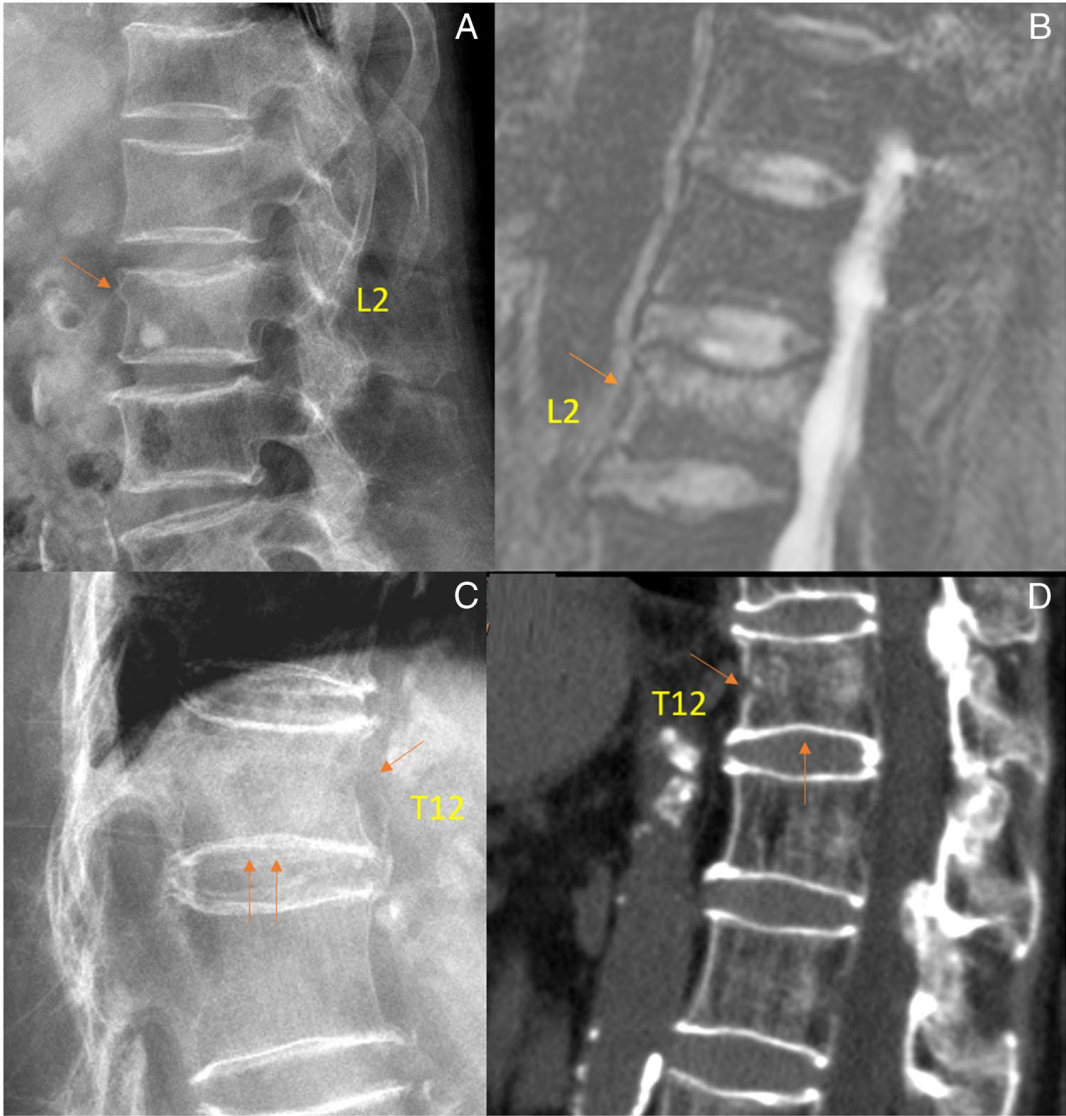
Spine imaging of an 88-year-old woman (A, B) and a 90-year-old woman (C, D). Both had a recent low-energy trauma history. For the 88-year-old woman, lateral radiograph (A) shows upper endplate depression of L2, with anterior cortex buckling, and slight vertebral height loss. Magnetic resonance imaging (B, T2-weighted fat suppressed image) shows L2 endplate depression and bone marrow edema. For the 90-year-old woman, lateral radiograph (C) shows indentation of the anterior cortex of T12 (arrow) and lower endplate depression (double arrow). CT shows a fracture of the anterior cortex of T12 (arrow) and slight inferior endplate depression (arrow). Note both involved vertebrae (L2 and T12) have a limited extent of height loss (< 20%). Reproduced with permission from Du and Wáng. Osteoporos Int. 2022;33:1569–1577
